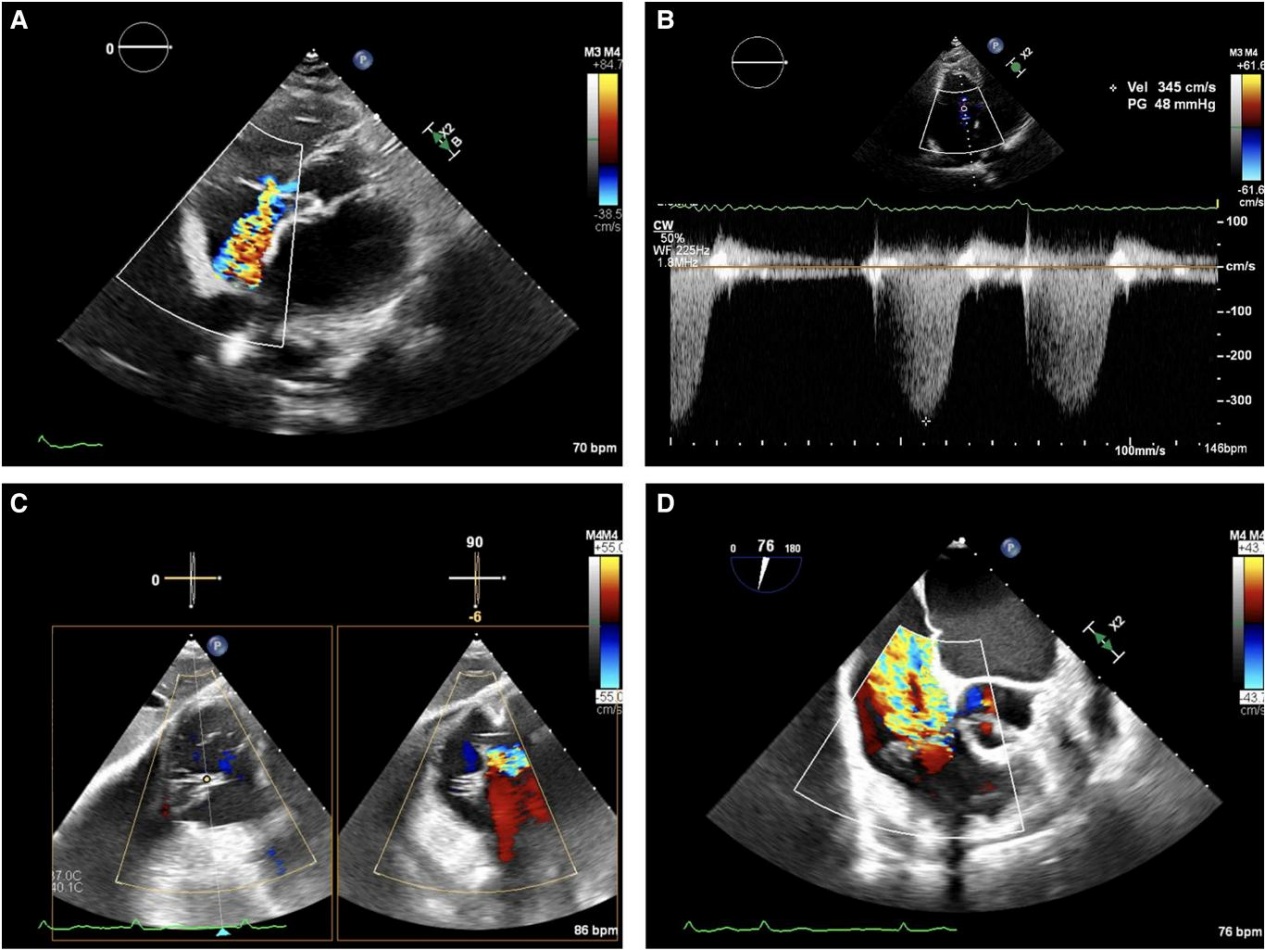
Transthoracic and transoesophageal echocardiography on admission. (*A* and *B*) Transthoracic echocardiography by apical five-chamber view showed severe tricuspid regurgitation, with tricuspid regurgitation pressure gradient of 48 mmHg. Transgastric (*C*) and mid-oesophageal (*D*) short-axis view at transoesophageal echocardiography confirmed severe tricuspid regurgitation.
